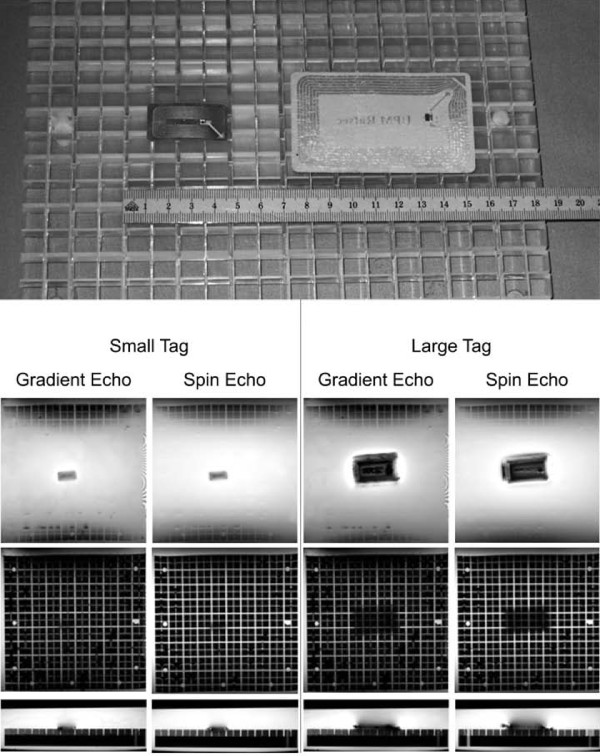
Quantitative image artifacts. Both RFID tags shown on the top have been placed one after the other in the middle of the plastic grid, immersed in 1 g/L CuSO4 solution. Gradient echo and Spin echo sequences as described in the ASTM Standard F2119-01 have been performed. The upper row shows MR images of a 3 mm-thick slice through the tags, the middle row slices 5 mm below the tag and the last row shows an orthogonal slice through the tag. Signal void could be found only a few millimeters away from the tag. Reduced signal due to shielding of the RF field may produce darker shadows in slices up to 1 cm away.
